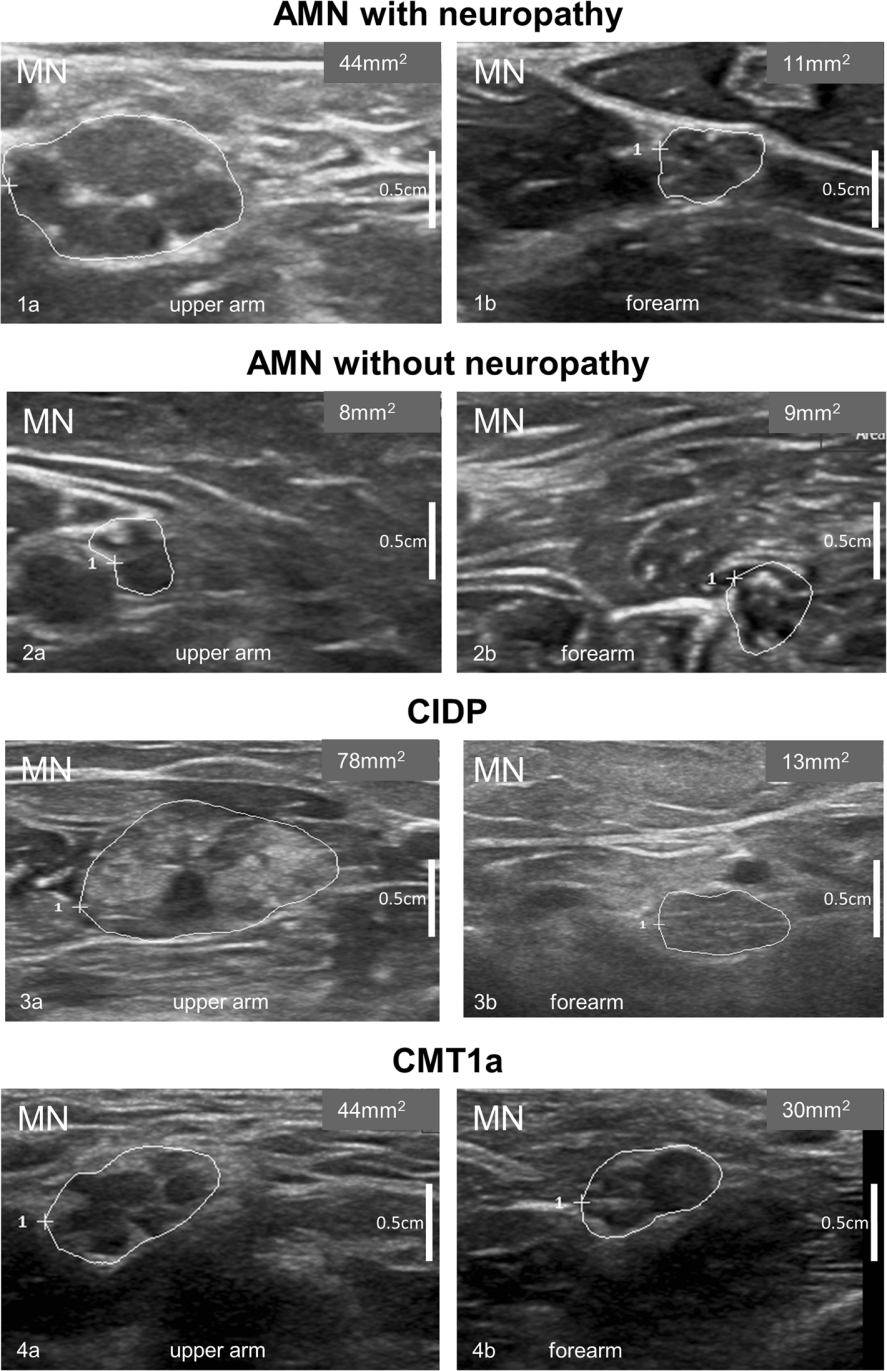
Representative ultrasound images of the median nerve (MN) in different diseases / disease states are shown per row. The left column (pictures labeled with an (a)) depicts cross sections of the upper arm (UA) and in the right column (b) cross sections of the forearm (FA). All ultrasound cross sections were recorded according to the UPSS protocol as previously described [8, 9]. All ultrasound pictures are presented at the same resolution and are therefore comparable in size (scale bar indicates 0.5 cm). In the first row (Ia&b) the inhomogenously enlarged median nerve of a patient with AMN and demyelinating polyneuropathy is seen (Class 1 according to [11]), with a cross-sectional area (CSA) of 44 mm^2^ in the UA (Ia) and 11mm^2^ in the FA – (Ib). Images of an AMN patient without neuropathy (Class 3, pictures IIa&b) are presented in the second row. The CSA of the MN was 8mm^2^ in the UA (IIa) and 9mm^2^ in the FA (IIb). CSA values of AMN patients without electrophysiologically proven peripheral neuropathy (PNP) correspond to normal values of healthy controls as published before [8]. For comparison purposes, we added representative pictures of a chronic inflammatory demyelinating polyneuropathy (CIDP) patient (Class 2) in the thirds row (IIIa & b) and of a Charcot-Marie-Tooth type 1a (CMT1a) patient (IVa & b) in the fourth row. The CIDP patient shows an inhomogeneously enlarged median nerve (78mm^2^ in the UA (IIIa) and 13mm^2^ in the FA (IIIb)) which is hyperechoic due to more perifascicular tissue. Nerve segments of the median nerve of a CMT1a patient (IVa & b) are in contrast homogeneously enlarged with 44mm^2^ in the UA (IVa) and 30mm^2^ in the FA (IVb) without significant changes in echointensity. The CSA in CMT1a equals the 3-4fold of known normal values in healthy adults
